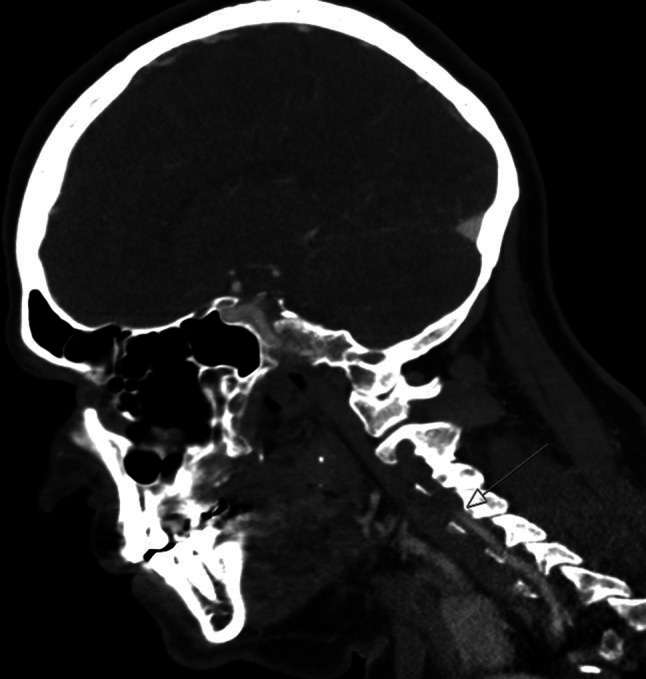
Computed tomography, sagittal view. *Arrow* shows occluded artery
